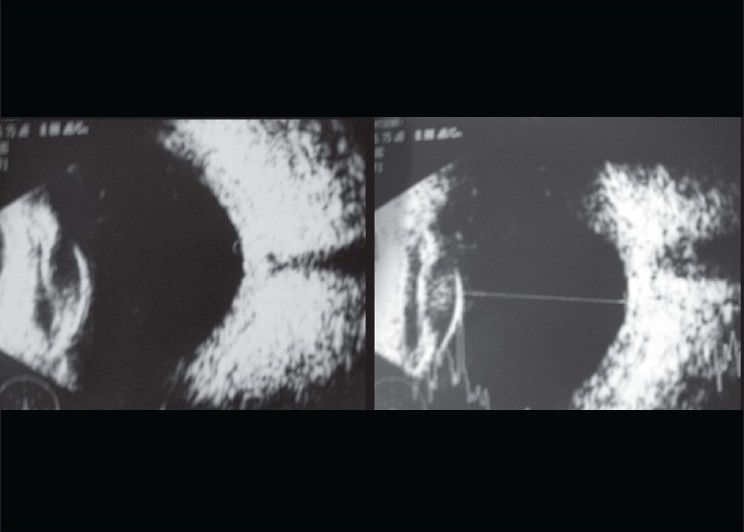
Ultrasound B-scan showed bilateral retinochoroidal thickening (arrows). Diagnosed as probable Vogt-Koyanagi-Harada's syndrome, she was treated with intensive topical betamethasone and mydriatics drops. She also received intravenous methyl prednisolone 1 g for five days followed by oral steroids
